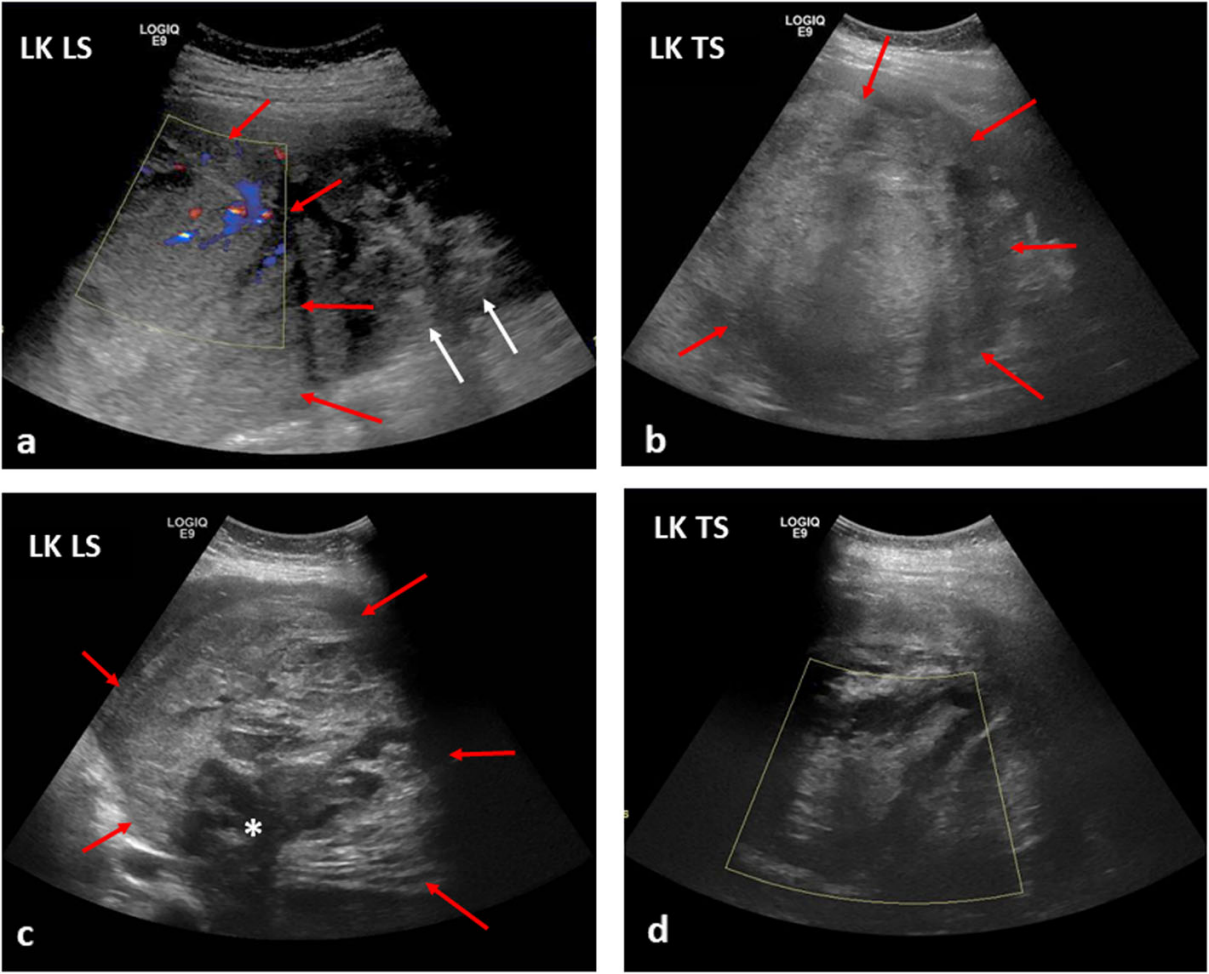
The same 13-year-old girl as in Fig. 18, with subsequent internal haemorrhoage within a left-sided Wilms’ tumour. a, b At diagnosis, the initial longitudinal (LS) and transverse (TS) ultrasound images of the left kidney (LK) reveal a large echogenic mass (red arrows) in the upper pole of the kidney, with some preserved renal tissue in the lower pole (white arrows). Some scant internal colour Doppler flow is noted within the mass. c, d A month later, after a sudden drop in the haemoglobin levels accompanied with intense abdominal pain, the ultrasound images of the left kidney reveal a larger, more heterogenous mass (red arrows) with some internal necrosis (white asterisk) and no internal colour Doppler flow in keeping with a large haematoma. It was not possible to clearly delineate the left renal vein at either the initial or subsequent ultrasound studies
